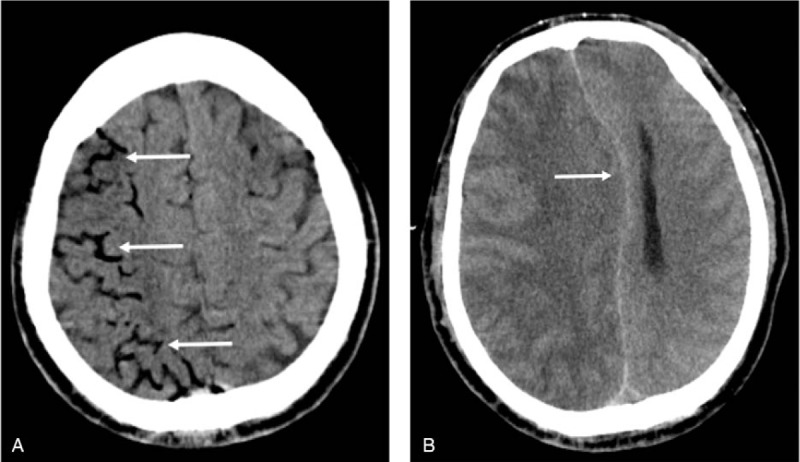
(A) Right middle cerebral artery branches with massive gas. (B) Cranial computed tomography showing large cerebral infarction in the right hemisphere and subfalcine herniation 48 hours after air embolism.
